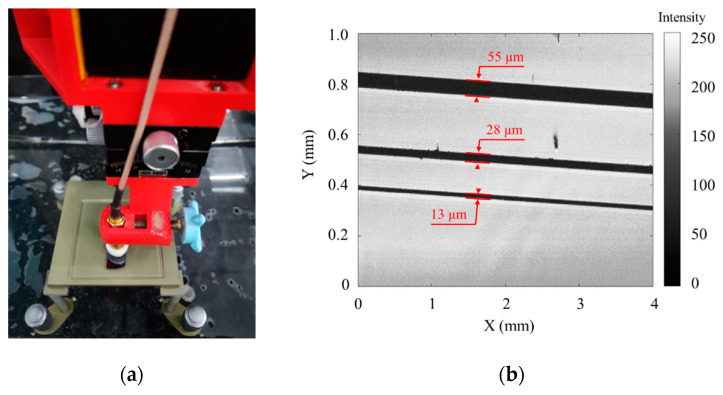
(a) Silicon wafer imaging testing. (b) The ultrasonic image of slots on silicon wafer.
